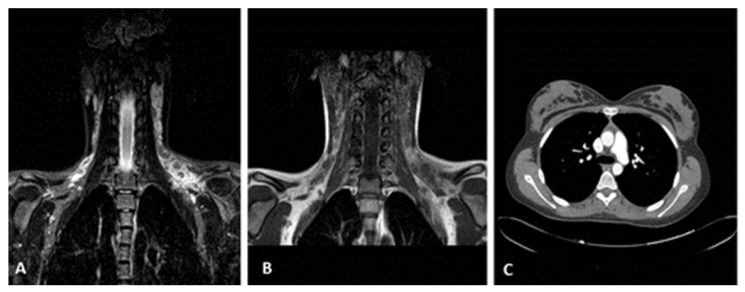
Radiological findings: (A,B) MRI in the cervical and supraclavicular areas, mainly on the left; multiple lymph nodes are observed that converge in some areas. Some of them, being rounded off, show an intermediate signal on the T1 sequence and a low signal on the STIR, while on the diffusion sequences, they show a restriction. The perilymph node tissue shows an intense high signal on fat suppression sequences to the left, while on T1 sequences, it shows inhomogeneous high signal and increased diffusion. (C) CT scan of the thorax and abdomen: no obvious pathological findings; a number of lymph nodes up to 7 mm in diameter are observed paraaortically and in the mesentery. Central tracheobronchial tree free, no pathologically sized lymph nodes in the hilar and mediastinum.
